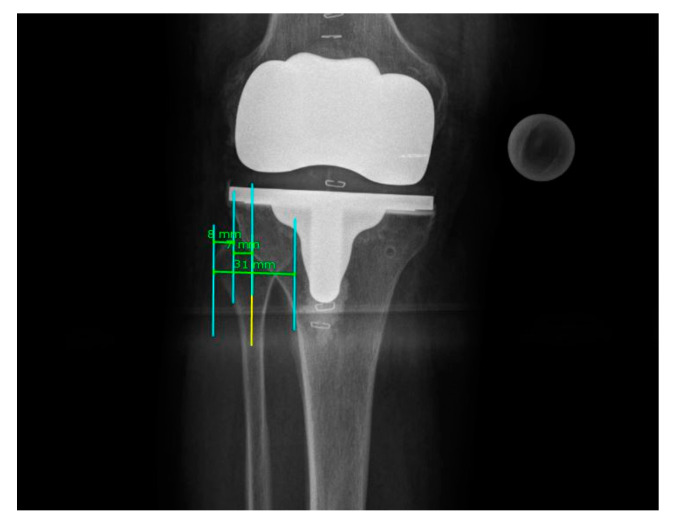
Detail of rotational analysis of a full-leg weight-bearing radiograph using the proximal tibio-fibular overlap.
